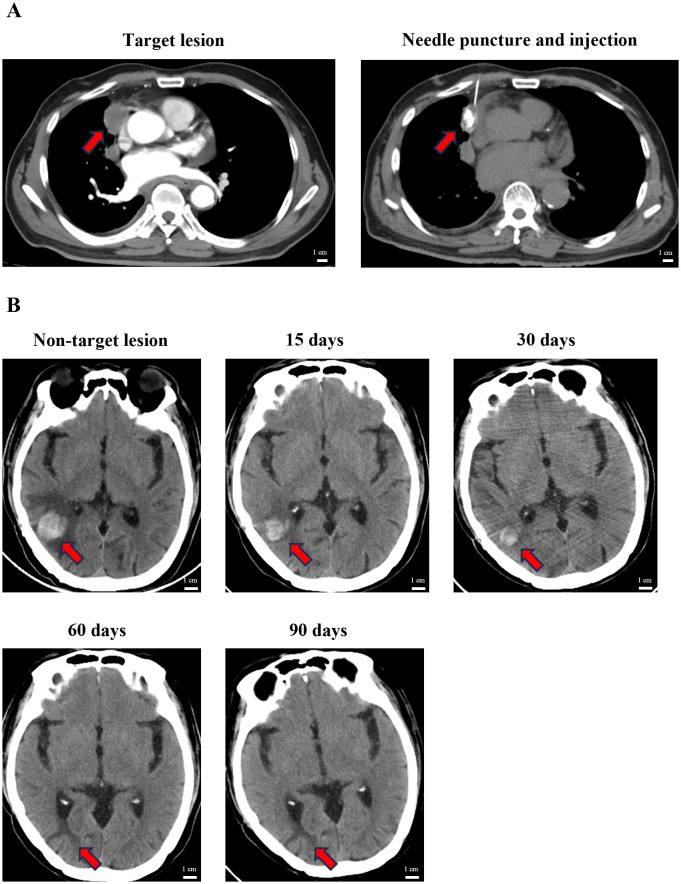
CT-guided intratumoral injection of the primary lung lesion and serial radiological follow-up of the untreated brain metastasis. (A) CT images of the target lung lesion before treatment (red arrow) and during percutaneous needle puncture and intratumoral injection (red arrow). (B) Serial CT images of an untreated non-target brain metastasis in the right occipital lobe, demonstrating progressive regression at baseline (pre-treatment) and at 15, 30, 60, and 90 days post-treatment (red arrows).
